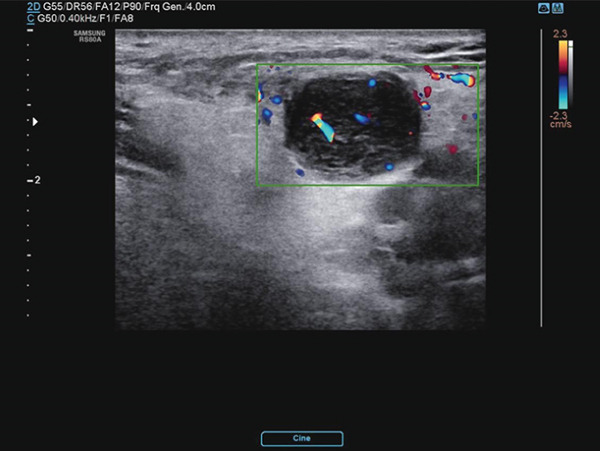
Ultrasonic manifestation showing the heterogeneous lesion with visible blood flow signals.
